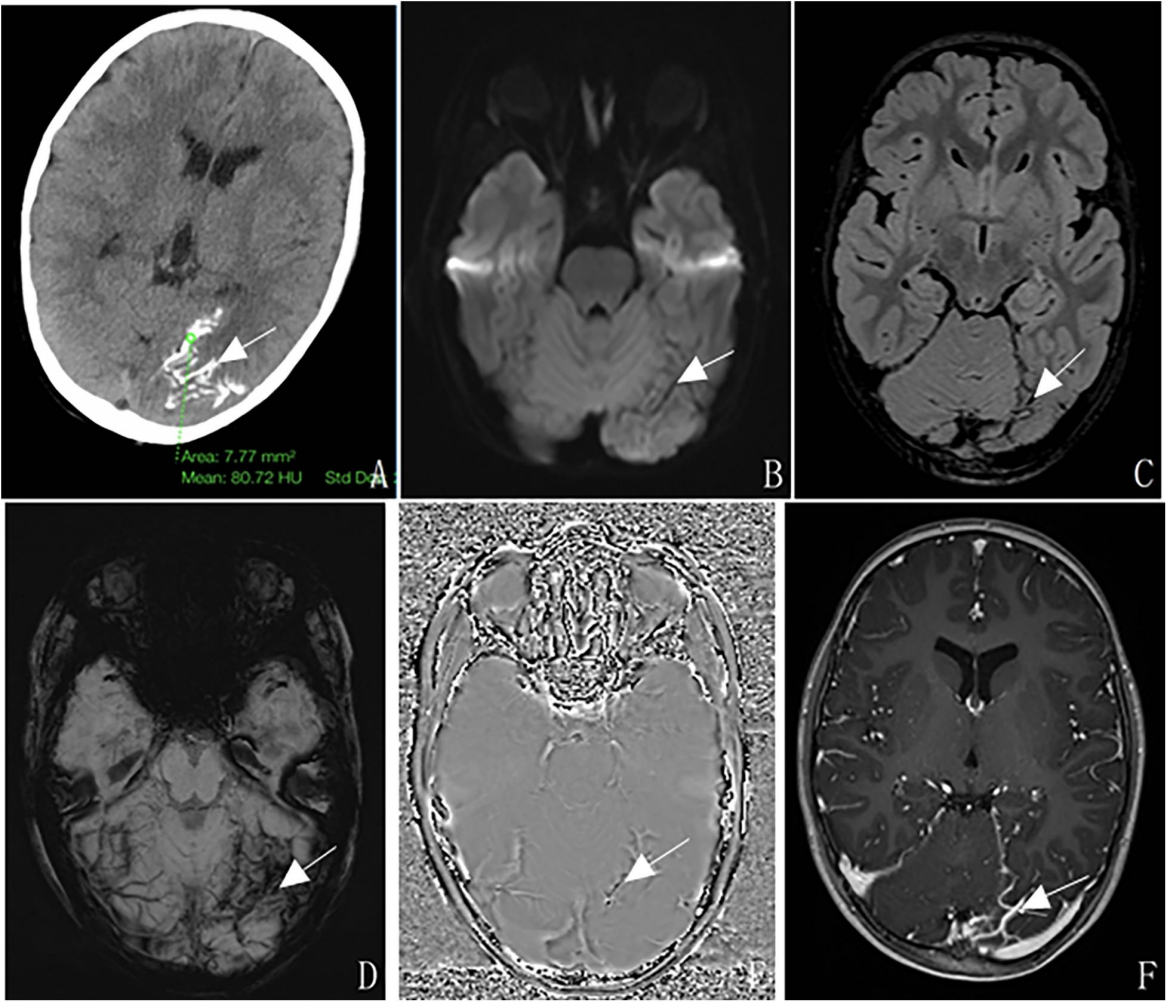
The patient is a 5-year-old male with Sturge-Weber syndrome type III. CT shows multiple tortuous vascular-like calcifications along the sulcus course of the left occipital lobe (A, arrow). MRI shows multiple tortuous strip-shaped low signals on DWI (B, arrow) and FLAIR (C, arrow); SWI images (D, arrow) and phase images (E, arrow) show multiple strip-shaped low signals in the left occipital lobe; After enhancement, there was a significant increase in tortuosity of the left occipital leptomeningeal vessels with enhancement (F, arrow).
